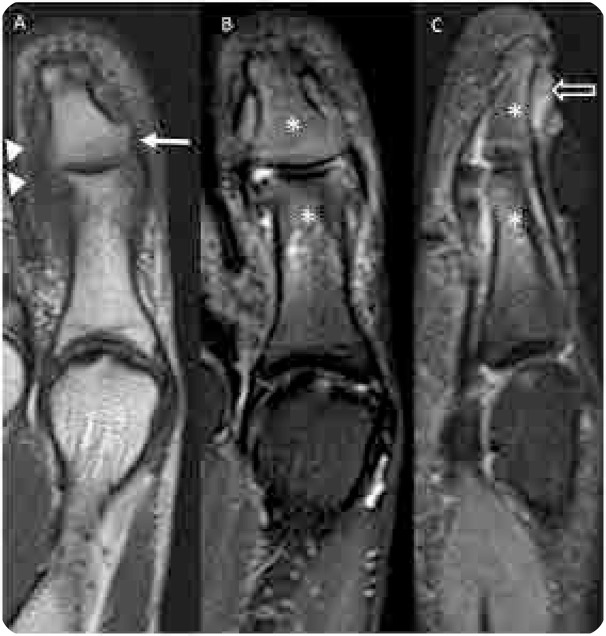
(right). Magnetic resonance imaging of the hallux. (A) Coronal T1-weighted image showing periarticular erosions (arrow) and soft-tissue swelling with intermediate to low signal intensity (arrowhead). Coronal (B) and sagittal (C) fat-suppressed proton density-weighted images depicting extensive bone-marrow oedema in the proximal and distal phalanges of the hallux (asterisks) related to the extensor and flexor enthesis, and oedema and thickening of the nail bed (open arrow in C).
